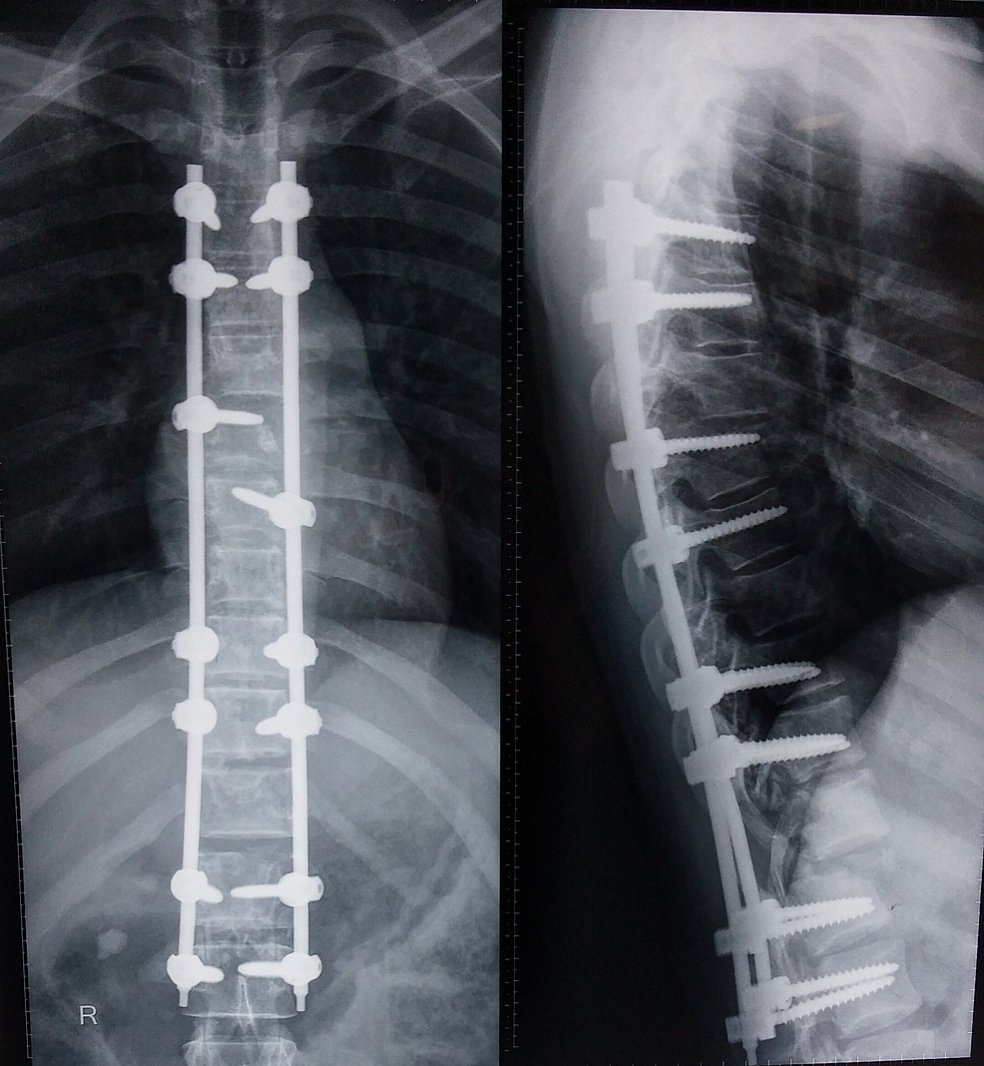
Postoperative radiograph following reduction and long-segment fixation
